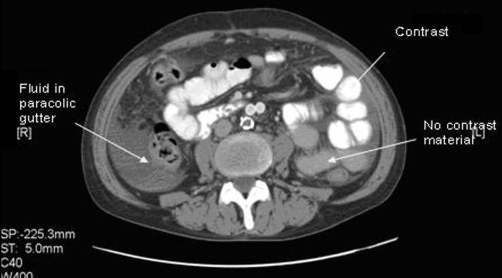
CT abdomen with and without contrast showing differential contrast distribution.
